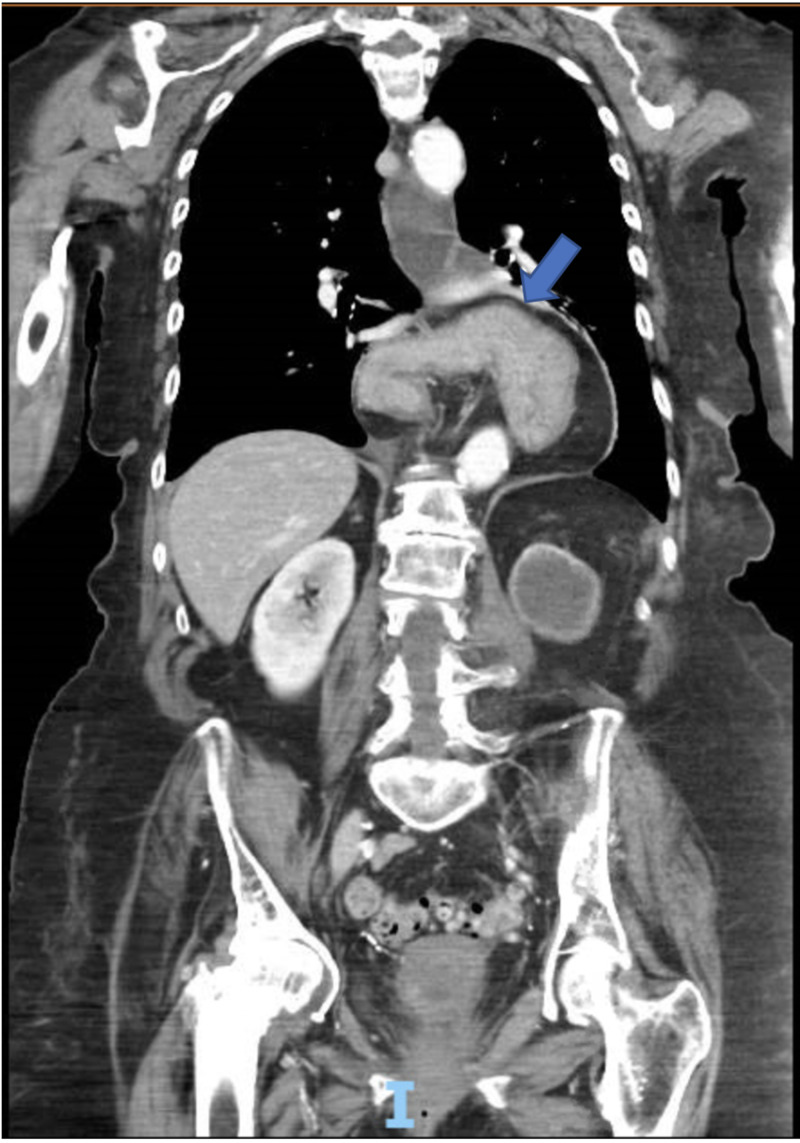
CT of the abdomen (axial view) showing organo-axial malrotation of the stomach.
